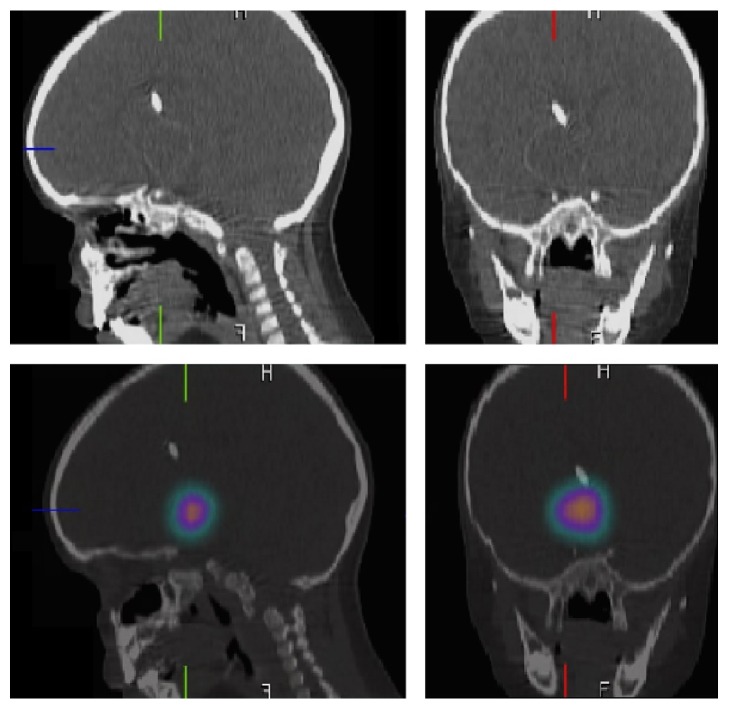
Postinjection Bremsstrahlung imaging by brain Single-Photon Emission Computed Tomography (SPECT). Brain SPECT coregistered with a computed tomography, showing a focal area of intense uptake in the suprasellar region, matching the shape of the cyst.
